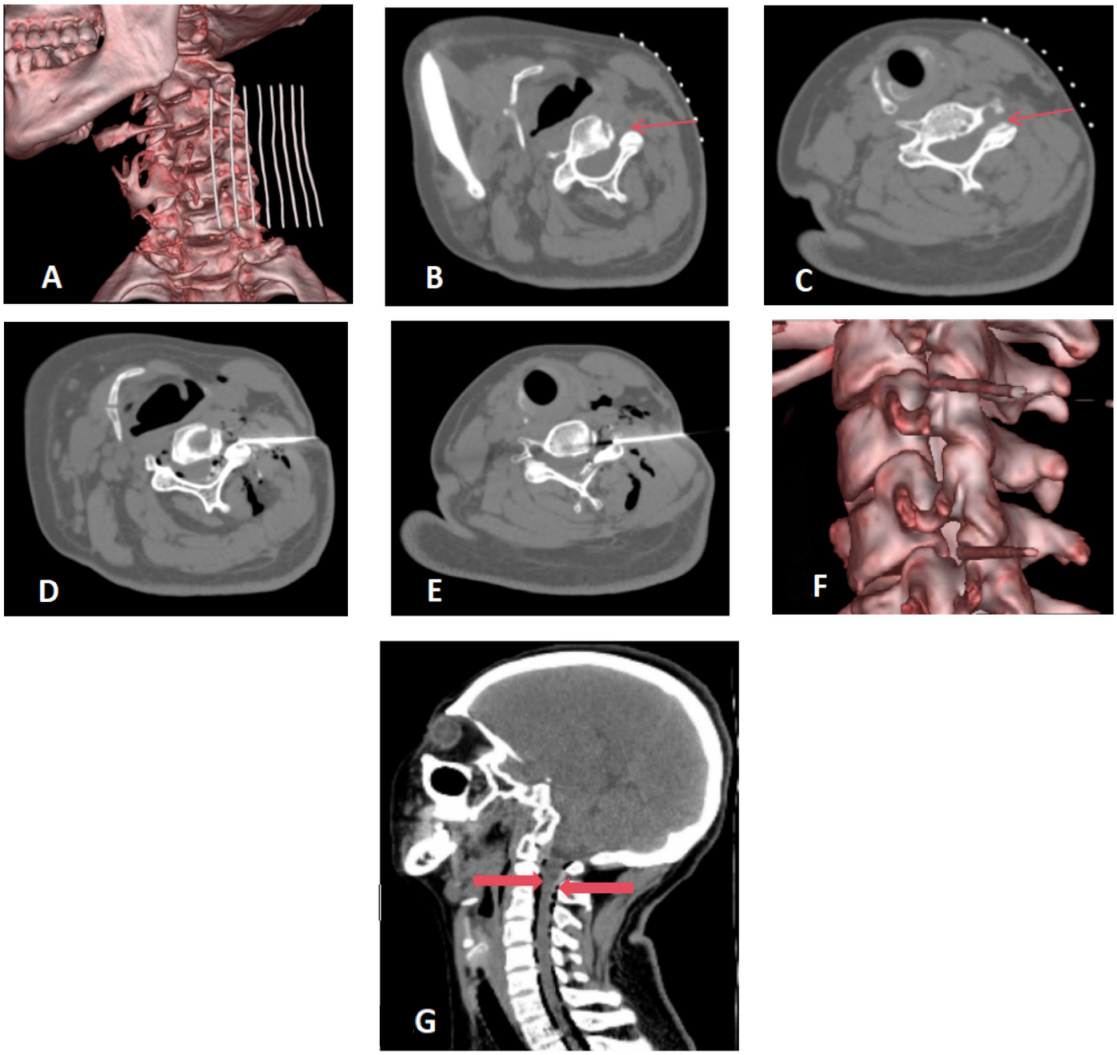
(A–C) Preoperative positioning: the patient was positioned supine on the operating table, with the head turned towards the contralateral side. A metal grid marker was strategically placed on the neck to facilitate precise targeting. The cervical anterolateral approach was employed, focusing on the articular pillar surfaces at the C3/4 and C5/6 levels. These target areas were distinctly marked with fine red arrows for clear visualization. (D–F) Needle placement and injection. The needle was precisely inserted along a pre-established pathway targeting the surface of the superior articular process. A therapeutic mixture consisting of 10 mL of ozone blended with physiological saline, complemented by 1 mL of contrast agent, was meticulously injected at each designated site. Virtual Reality (VR) reconstruction techniques were utilized to confirm the accurate positioning of the needle tip at the level of the intervertebral foramen. (G) Postoperative CT scan reconstruction. The sagittal view of the cervical spine after surgery exhibits a satisfactory dispersion of ozone throughout the spinal canal. Gas shadows, clearly surrounding the dura mater, are marked by thick red arrows. There are no indications of hemorrhage detected in the visualized areas.
